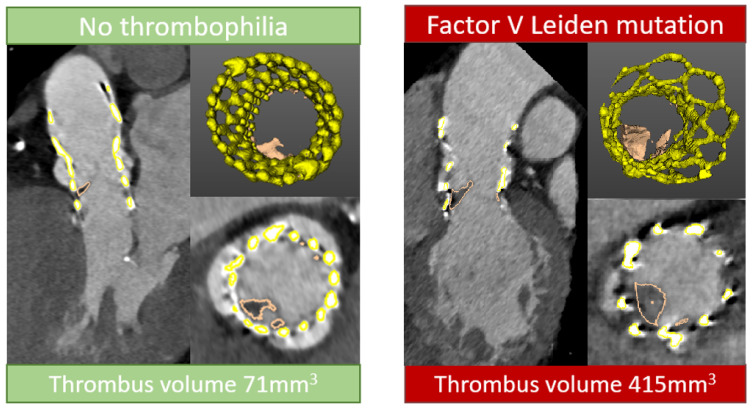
Quantitative thrombus characterization from computed tomography angiography.
